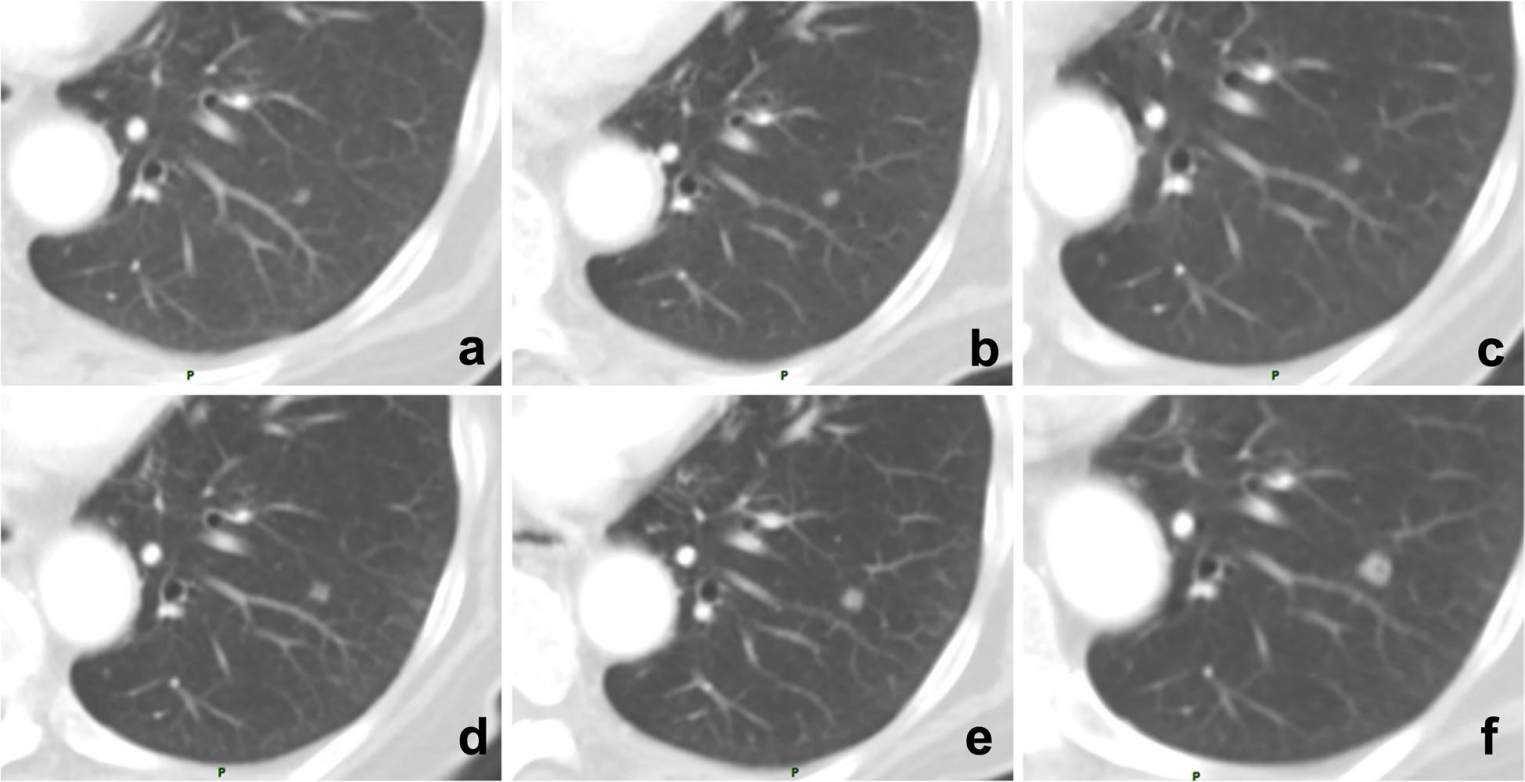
A 71-year-old man underwent multiple follow-up visits after colon cancer surgery. a–f Images showed a small nodule in the lower lobe of the left lung, but the radiologist only compared the current CT image with that of the last examination and reported no changes. By comparing each CT image, the gradually enlarging nodule was recognized, and follow-up imaging confirmed it as a metastatic tumor
